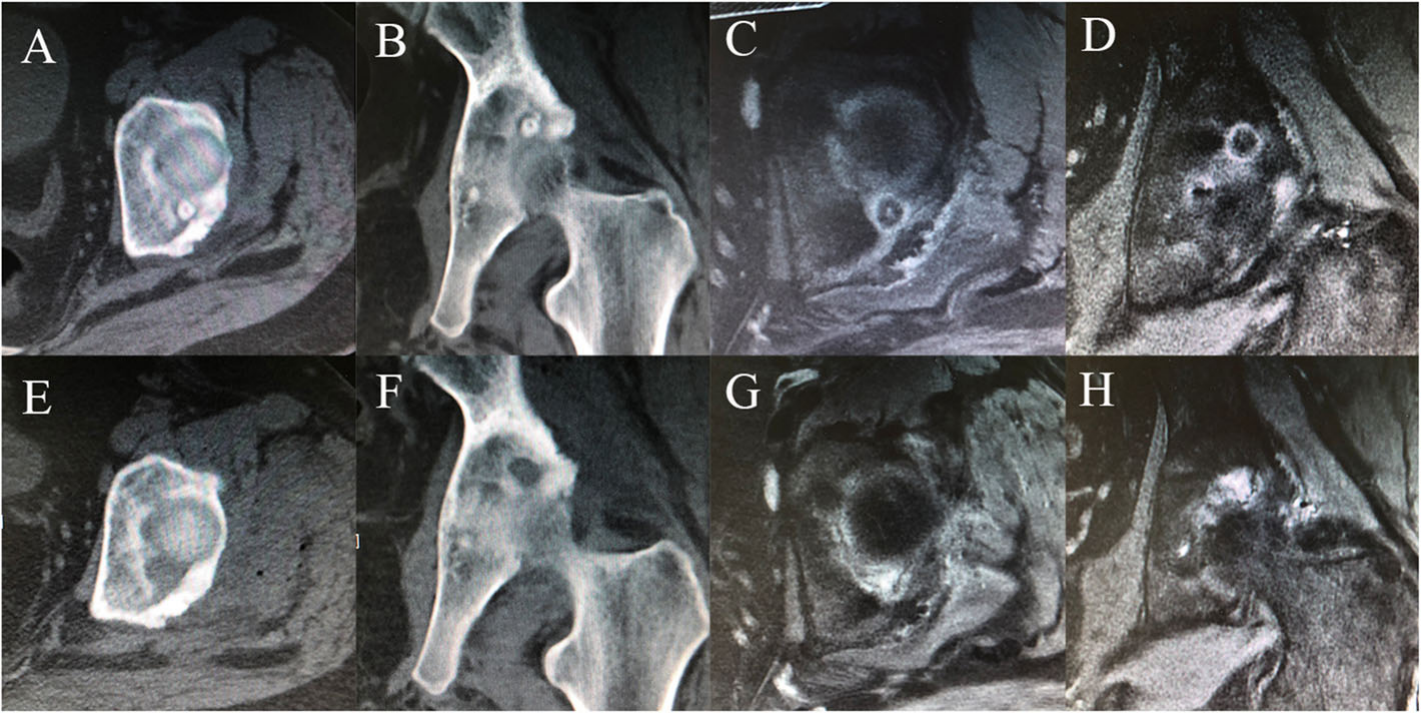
Preoperative and postoperative CT and MRI of one patient diagnosed with OO of the acetabulum in zone 4. A-D. Preoperative axial CT, coronal CT, axial MRI and coronal MRI showed the location of OO. E-H. Postoperative axial CT, coronal CT, axial MRI and coronal MRI showed the excision of OO
